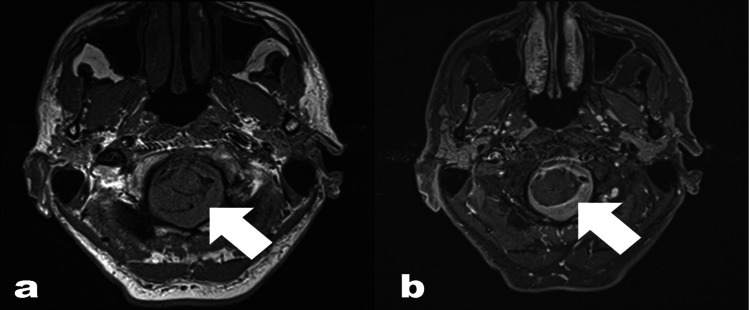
(A): Axial non-contrast T1 weighted spin echo MRI image of the brain showing asymmetric, smooth, abnormal pachymeningeal thickening at the foramen magnum (arrow), more pronounced on the left side. (B): Axial post-gadolinium contrast T1 weighted spin echo MRI image at the same location demonstrating avid enhancement of the pachymeningeal thickening (arrow).
